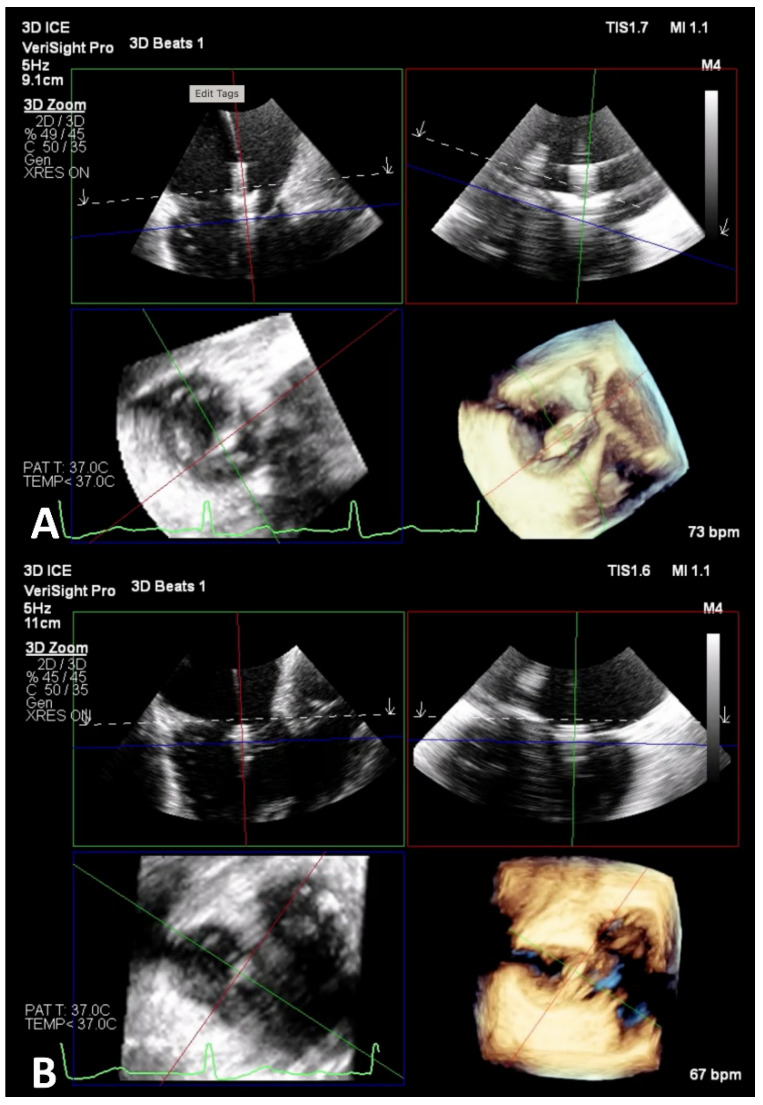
Adjunctive 3D ICE imaging during Triclip procedure. Panel (A): The proximity of the ICE catheter to the TV plane allows for a better visualization of the TV leaflets. Panel (B): 3D ICE is a supplementary imaging modality that is helpful during leaflet grasping and determining appropriate leaflet insertion. ICE, intracardiac echocardiography; TEE, transesophageal echocardiogram; TV, tricuspid valve.
